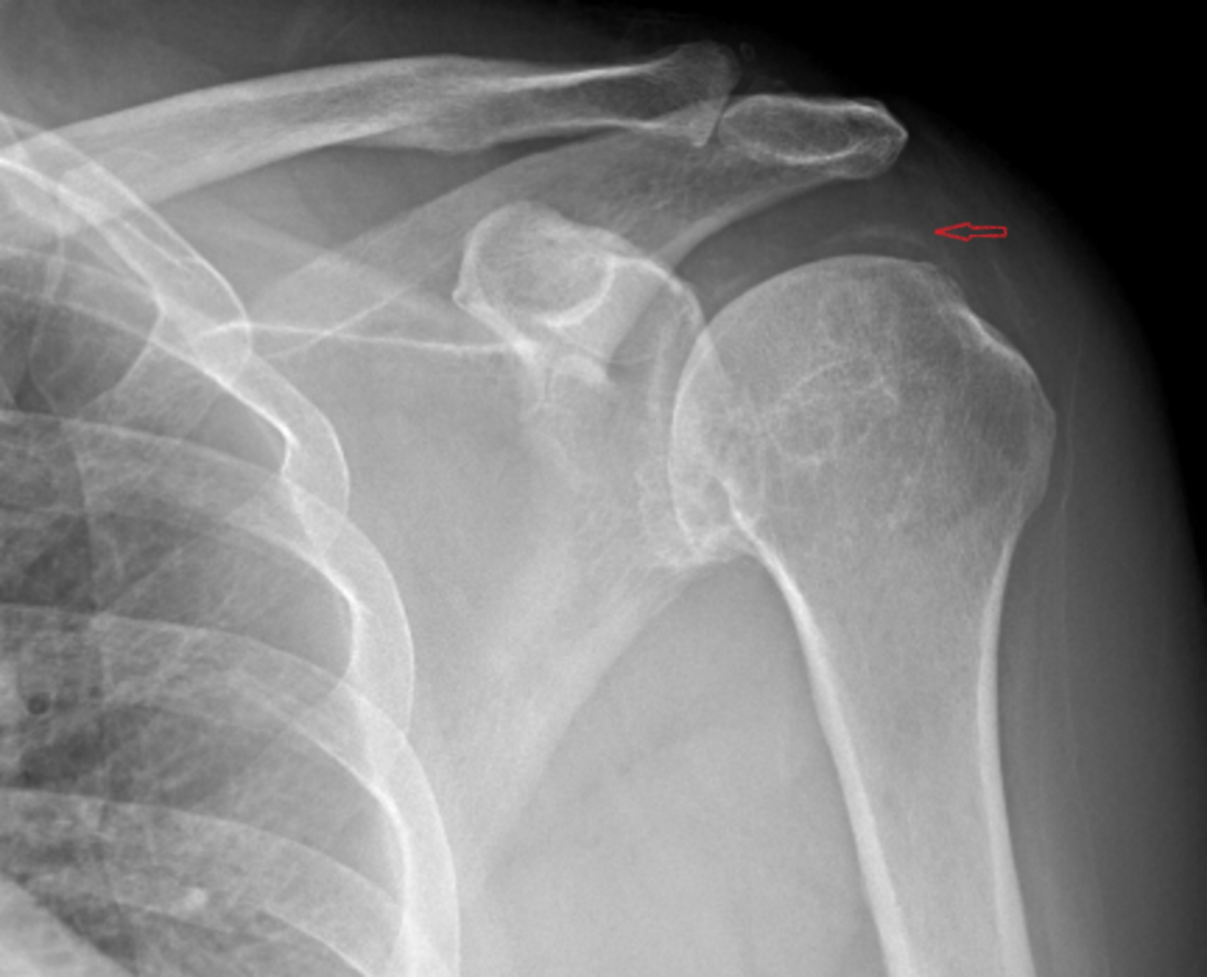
Radiograph showing chondrocalcinosis of the glenohumeral joint space The arrow identifies area of chondrocalcinosis of the glenohumeral joint space.
